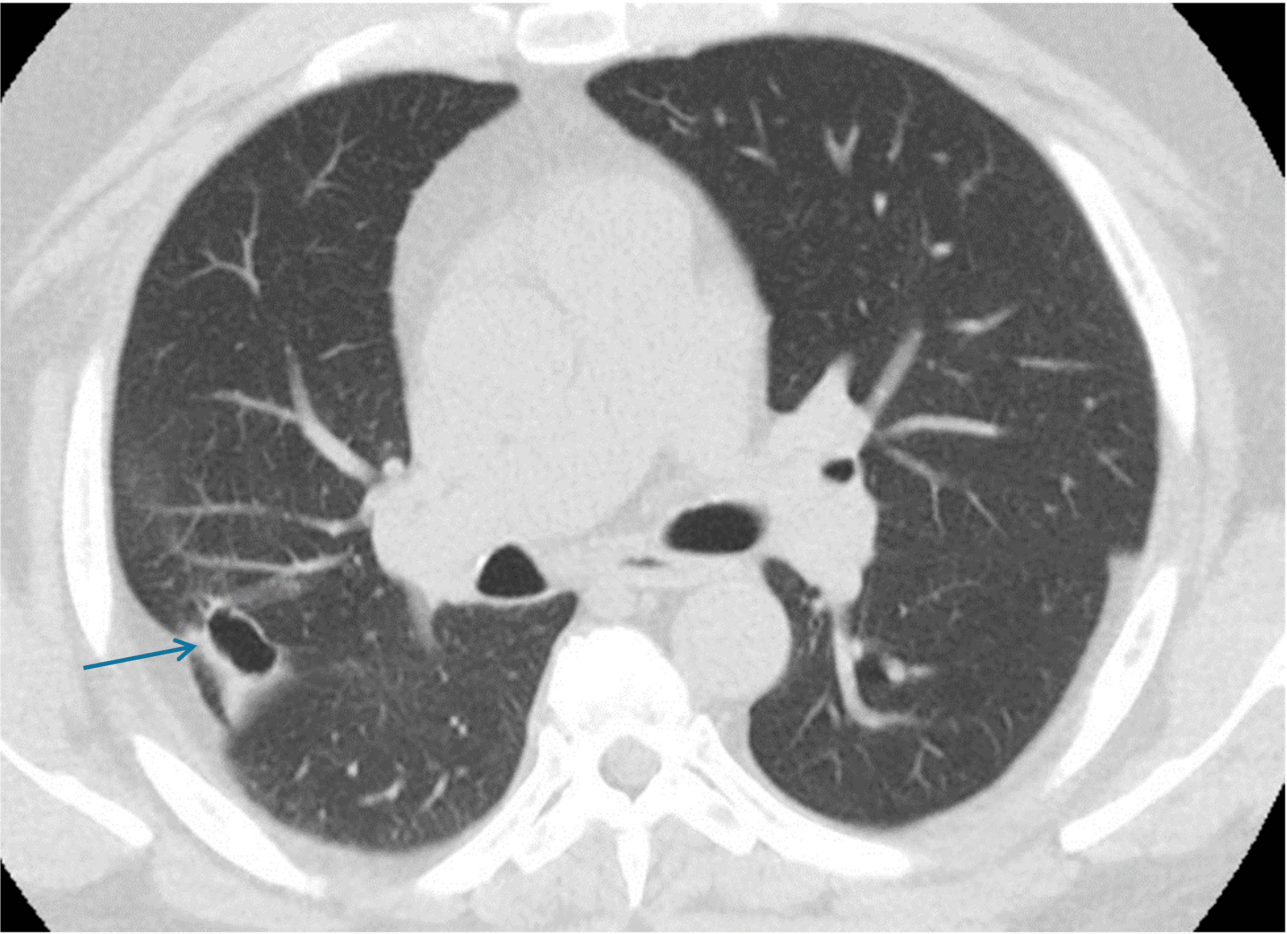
CT scan image at follow-up Persistent posterior right upper lobe (RUL) cystic structure.
